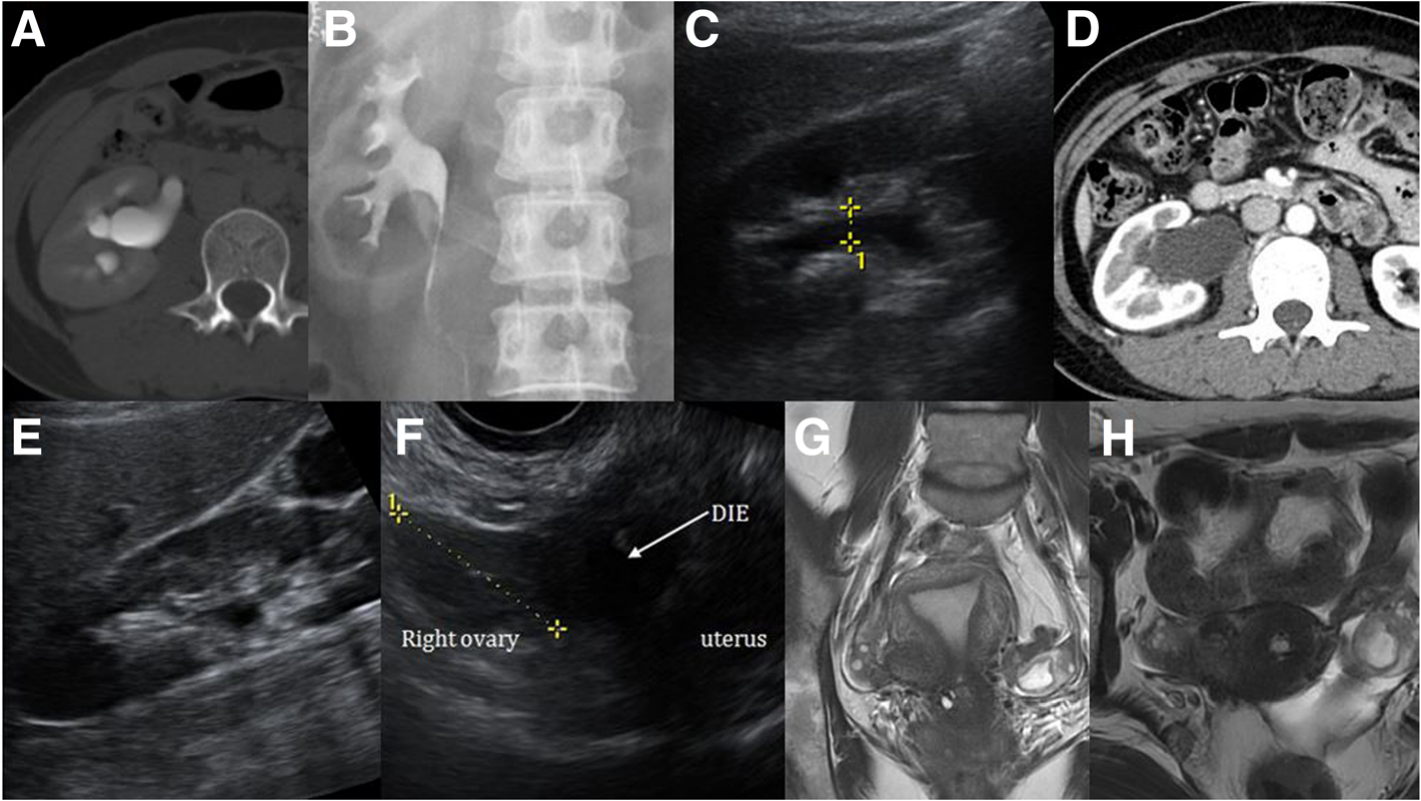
Computed tomography showed right hydronephrosis due to distal ureteral narrowing 4 years prior (a). After balloon dilation, the patient’s right hydronephrosis was relieved (b). Transabdominal ultrasonography (c) and computed tomography (d) demonstrated right hydronephrosis due to narrowing of the distal ureter at the start of menstruation. After menstruation, hydronephrosis was relieved (e). Transvaginal ultrasonography revealed uterine deviation to the right and a nodular lesion at the right uterosacral ligament (f). Magnetic resonance imaging revealed suspected deep infiltrating endometriosis near the right uterus and ovary, with adhesions around the distal ureter (g and h)
